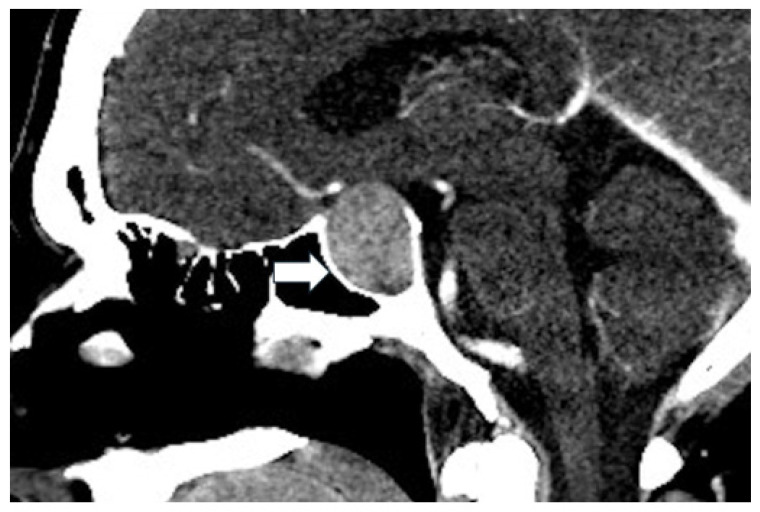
Sagittal CT image demonstrating enlargement of the ST (arrow) with inferior bulging of the ST floor in the sphenoid sinus, corresponding to the adenoma shown in Figure 2. The cortical bone remains intact.
